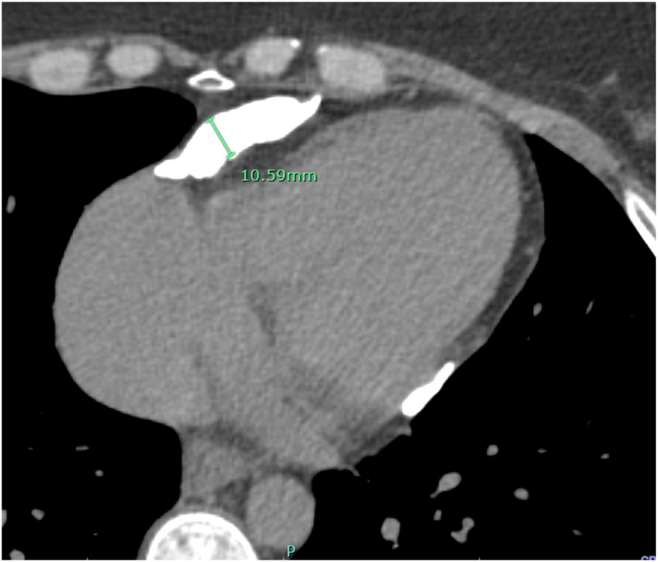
Case 3. Chest computed tomography demonstrates significant pericardial calcification, especially at the lateral wall of the pericardium up to 10.6 mm (*green line*).
